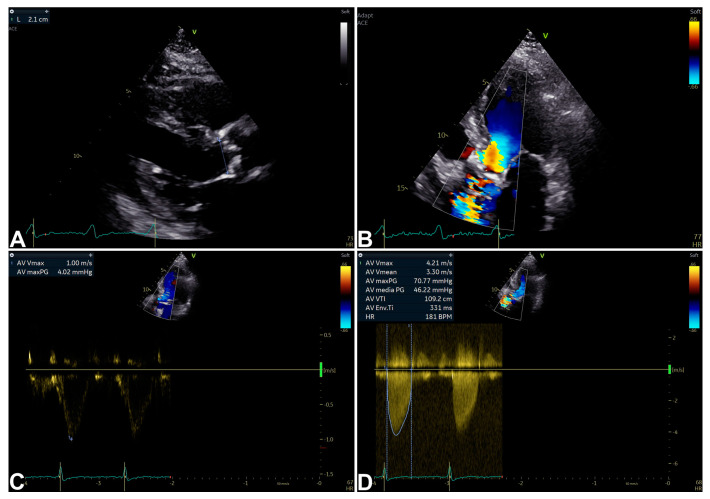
An example of SAS diagnosis by echocardiographic two-dimensional (2D) and Doppler data [9,10]. Two-dimensional parasternal long-axis view showing inner edge to inner edge measurement (2.1 cm) of aortic valve annulus at mid-systole (A); 2D apical five-chamber view with aortic transvalvular aliasing suggesting SAS (B); pulsed-wave (PW) Doppler (maximum velocity measured at 1.00 m/s) (C); and continuous-wave (CW) Doppler (maximum velocity measured at 4.21 m/s; maximum and mean gradients calculated result 70.77 mmHg and 46.22 mmHg, respectively) (D) measured from 2D apical five-chamber view diagnosing SAS.
